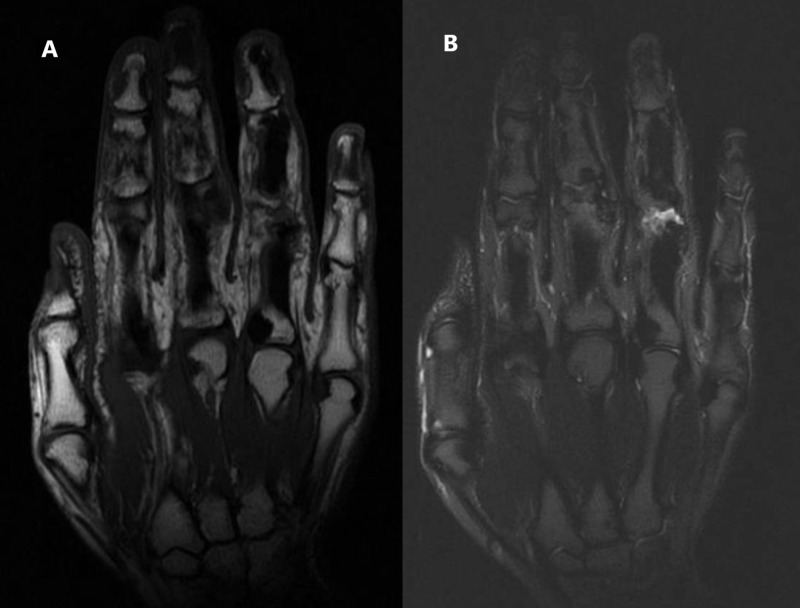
MRI of the right hand. A) T1-Weighted MRI. B) T2-Weighted MRI.
